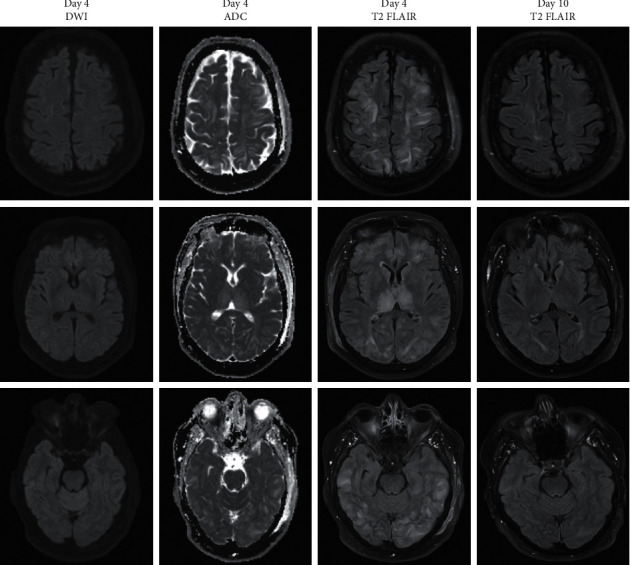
Sequences of MRI brain including DWI, ADC, initial FLAIR, and FLAIR on follow-up imaging, from left to right. Initial MRI (first three columns on the left) noted symmetric subcortical and cortical FLAIR hyperintensity involving the bilateral cerebral hemispheres including extensive involvement of the thalami and basal ganglia, without contrast enhancement. DWI was isointense, lacking diffusion restriction, and ADC was hyperintense, consistent with vasogenic edema. FLAIR sequence on repeat MRI (fourth column from the left) noted significant interval improvement, with mild residual hyperintensity in the parieto-occipital white matter.
